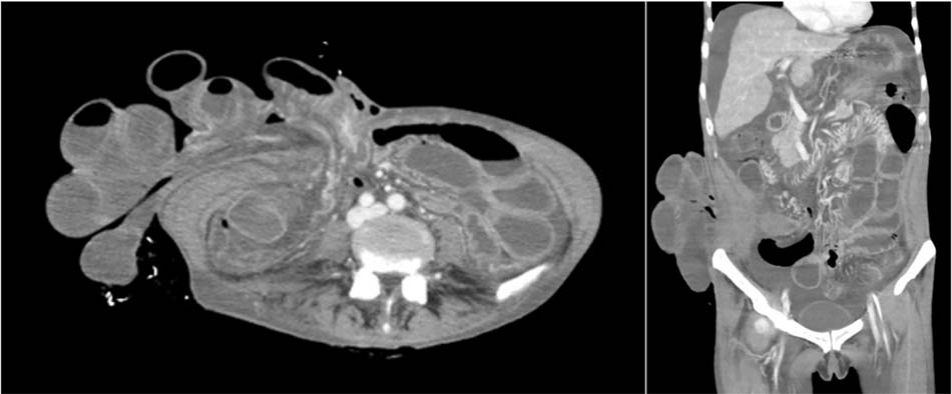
Abdominal computed tomography showing liver atrophy and intestinal evisceration due to ruptured umbilical hernia.
